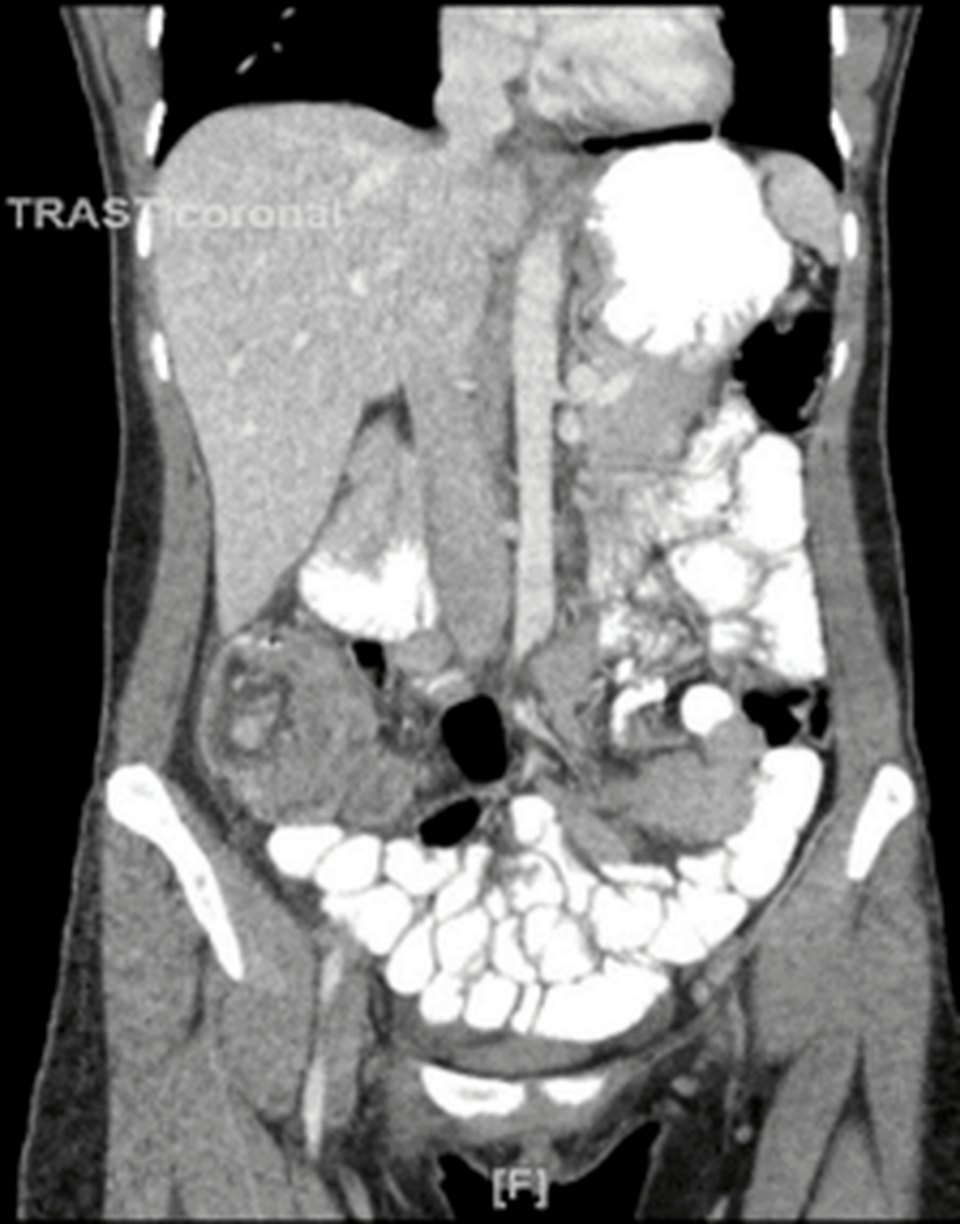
Coronal view of the CT scan of the abdomen with contrast showing non-distended small bowel loops and ileocecal intussusception.
